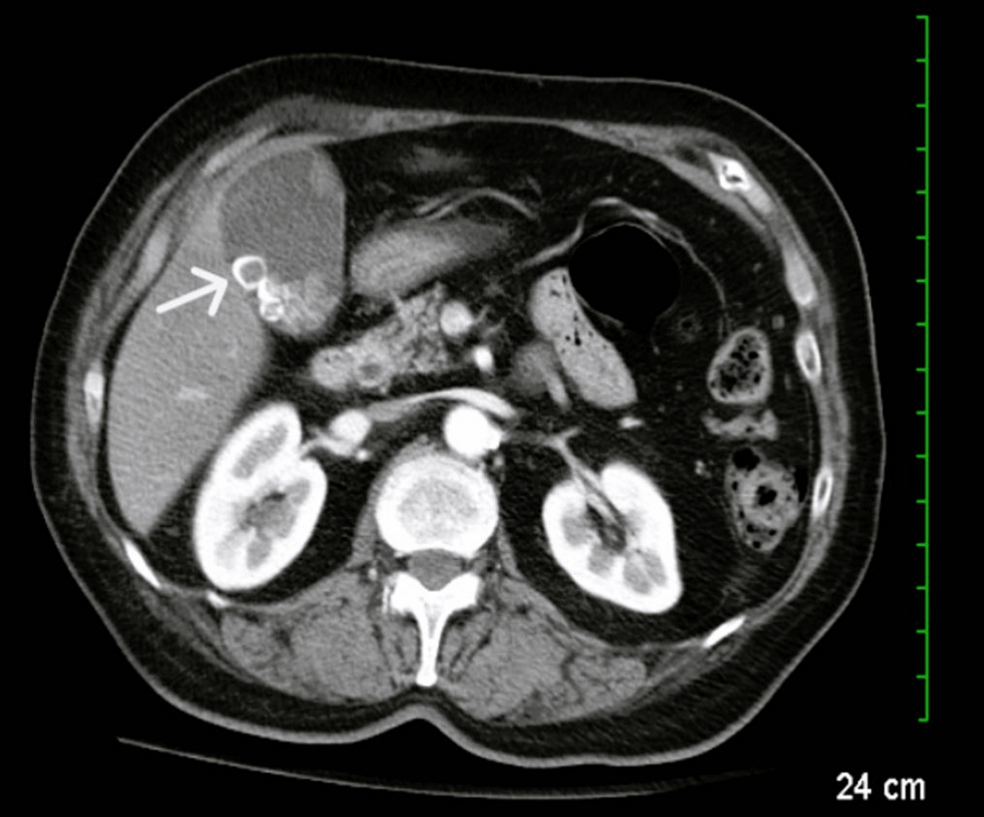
Abdominal CT demonstrating cholelithiasis (white arrow) CT: Computed tomography.
